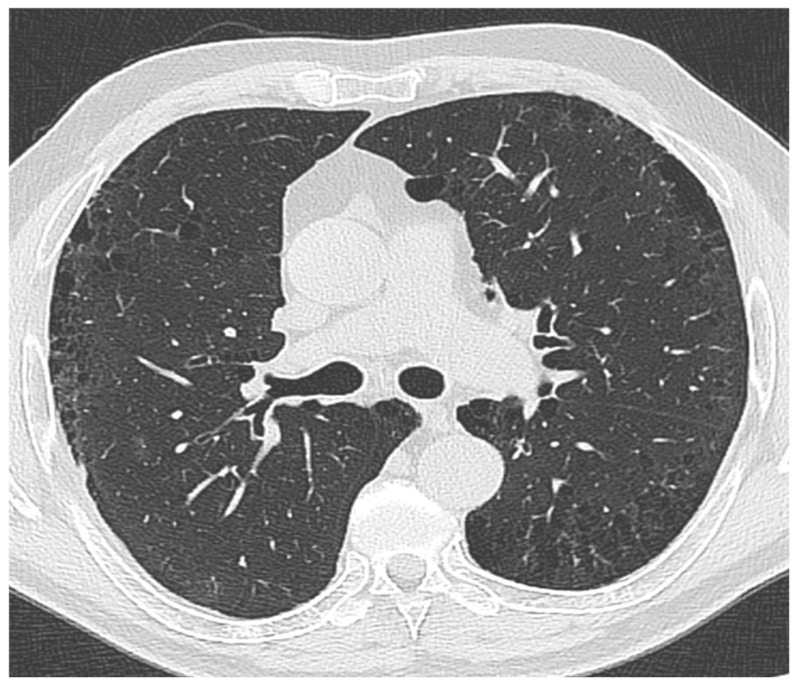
Thoracic computed tomography image of a patient included in the study demonstrating thin-walled, low-attenuation air spaces located predominantly in the subpleural regions, particularly in the upper lobes. These imaging findings are consistent with the radiological features characteristic of paraseptal emphysema.
